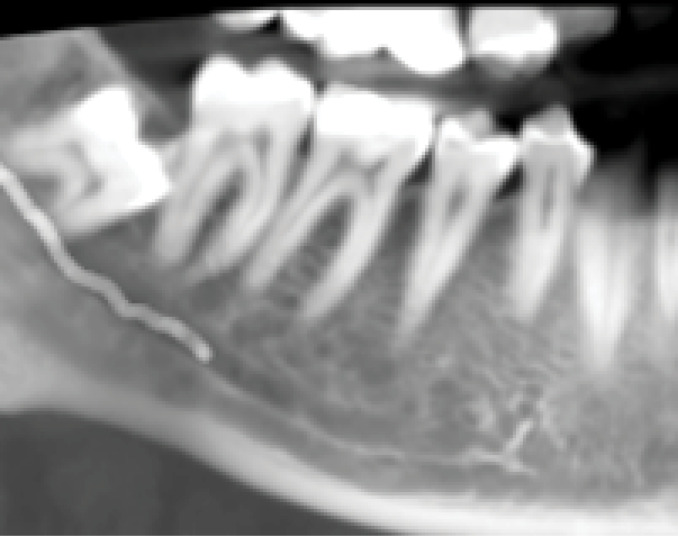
Alternatively, as already noted, an IMI may be placed into one or other of the molar root sockets, but it is helpful to know the mean buccal and lingual cortical bone thicknesses here. Our data showed that lingual cortical thicknesses were always greater than buccal ones and that all thicknesses generally increased progressively from the first molar mesial root socket to the distal root socket of the second molar. Such thin buccal bone would make the selection of implant diameter important to leave gaps (“jumping distances”) of ≥ 2 mm for hard tissue grafting to avoid excessive bone remodeling that could ultimately leave the buccal implant surface denuded of bone. In contrast, the mean crestal buccal bone thicknesses at second molars ranged from 0.64 mm to 3.22 mm for mesial roots, making it more likely that a larger diameter and shorter implant could be considered here compared to one placed in the mesial root of a first molar. We can agree with the approach suggested by Chen et al^7^ that if the patient wishes to have both mandibular molars replaced with immediate implants, a safe approach would be to use the ISB at the first molar but to place the second implant in the mesial root socket of the second molar. The measurements of buccal thickness taken at the mid-root positions were also significantly different between the two molar locations (1.81 MB first molar vs. 4.78 MB second molar and 2.33 DB first molar vs. 5.08 DB second molar), which might suggest that ridge preservation^15^ and delayed implant placement were being contemplated, second molar sites with their thicker buccal cortical plates could be less likely to need SPG (socket preservation grafting) since thicker buccal bone does lead to less buccal bone resorption.^16^ This paper builds on our previous studies, which discussed the relevance of CBCT measurements for immediate maxillary molar implantation^17^ and guidelines for IMI placement.^18^
